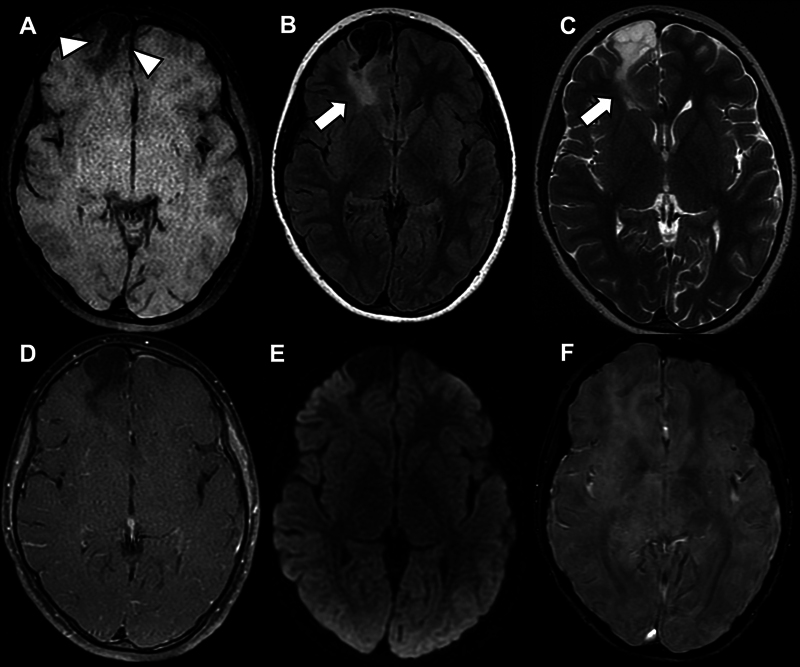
Non-contrast T1-weighted imaging (T1-WI) (
